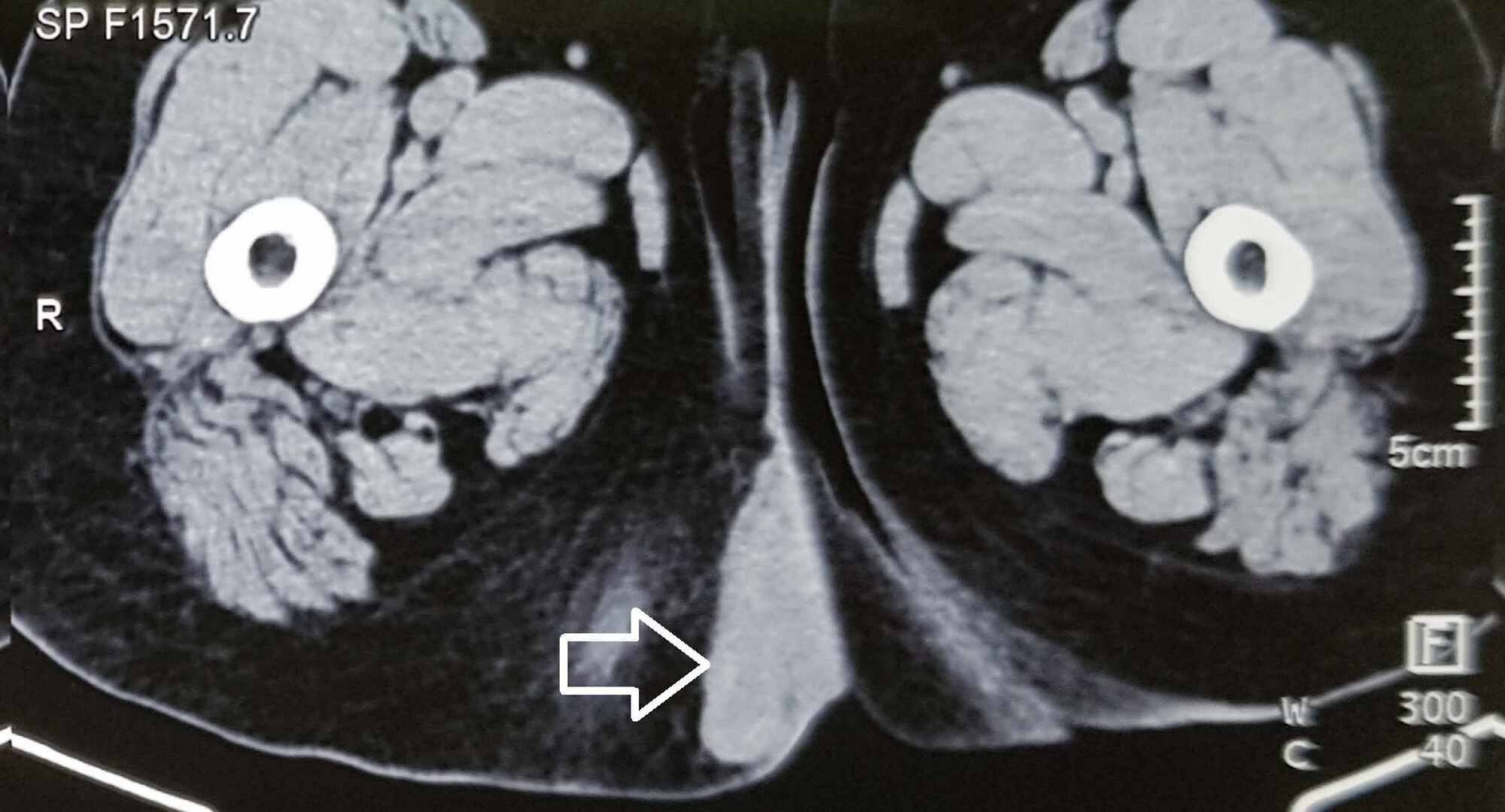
Computed tomography of the pelvis showing the perineal lesion suggestive of abscess formation (arrow).
